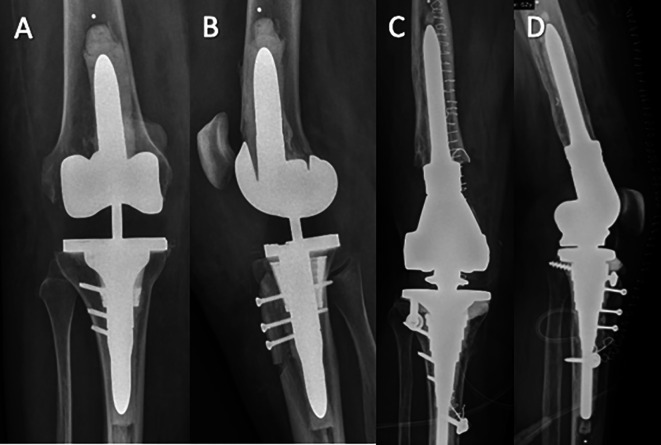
Postoperative radiographs: example of two different types of constraint. A, B: Varus Valgus Constraint implant; C, D: Rotating hinge. In both cases, TTO fixation was possible using screws despite tibial cone or sleeve.
